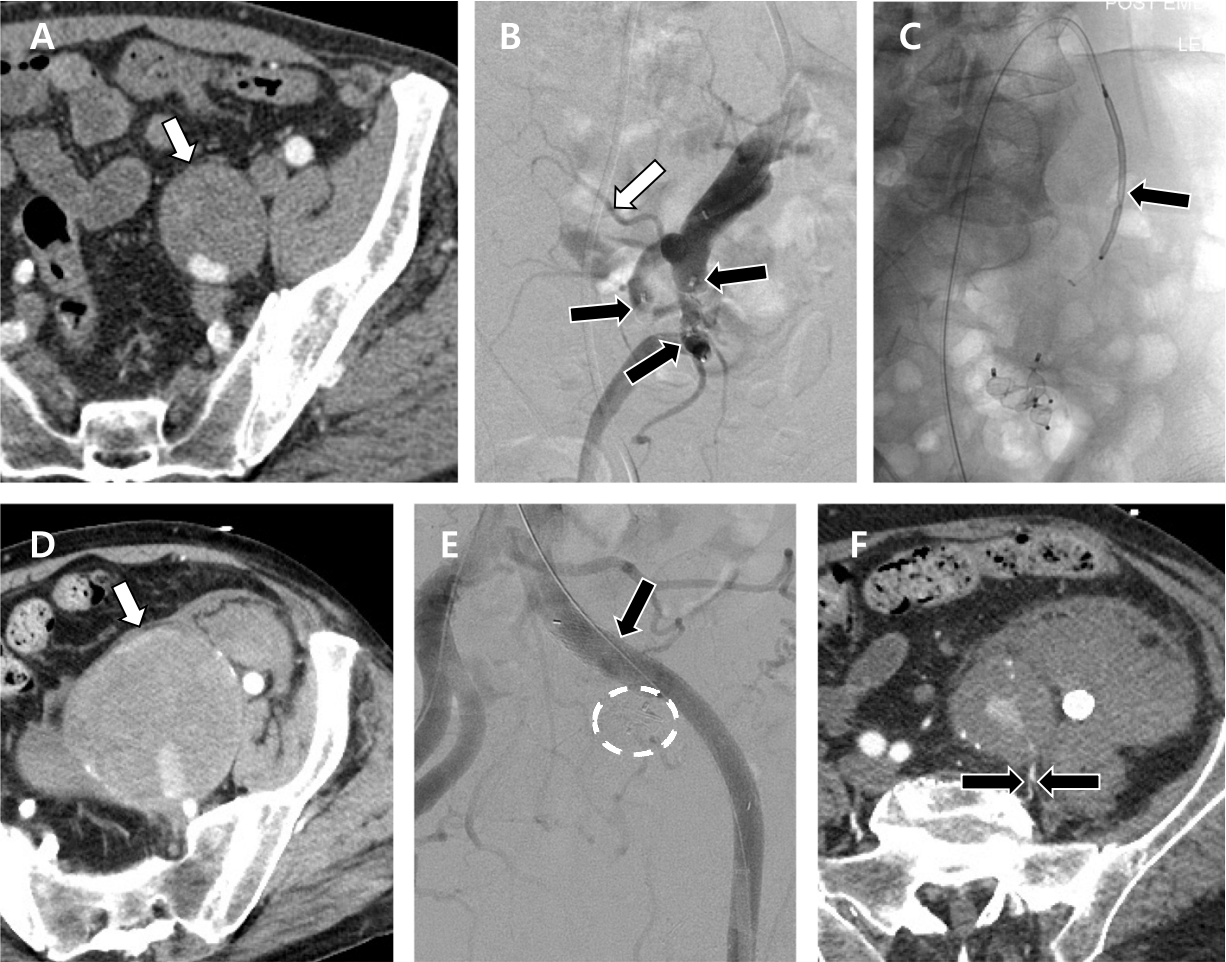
Ruptured IIA aneurysm in a 63-year-old male resulting from persistent type 2 endoleak. A Initial CT demonstrates an isolated IIA aneurysm (white arrow) measuring 42 mm in diameter. B Vascular plugs (black arrows) were placed distally to the bifurcation of the anterior and posterior trunks of the IIA. Not a prominent iliolumbar artery (white arrow) arising proximally to the embolization site. C A vascular plug (black arrow) was placed in the proximal segment of the IIA to interrupt the inflow. D The patient returned 5 years later with a ruptured aneurysm (white arrow). E An iliac stent-graft was deployed over the ostium of the IIA (black arrow) which had previously been embolized with a vascular plug (white circle). F Follow up CT acquired one week later demonstrates persistent endoleak associated with retrograde flow through a patent iliolumbar artery (located between two black arrows). The patient expired three weeks later from multiorgan failure
